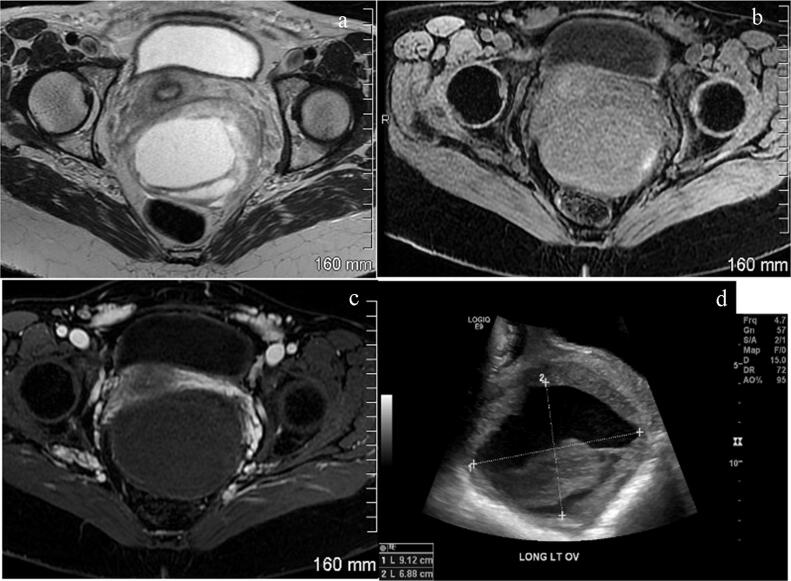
At 27 weeks gestation (a) T2-weighted MRI, (b) T1-weighted MRI, (c) T1-weighted MRI with contrast, demonstrate cystic mass with single septation and intrinsically T1 hyperintense fluid but no solid enhancing internal components following contrast, (d) At 34 week 3 day grayscale ultrasound image show mild continued growth of cystic lesion with solid appearing debris without internal blood flow on Doppler (not shown).
